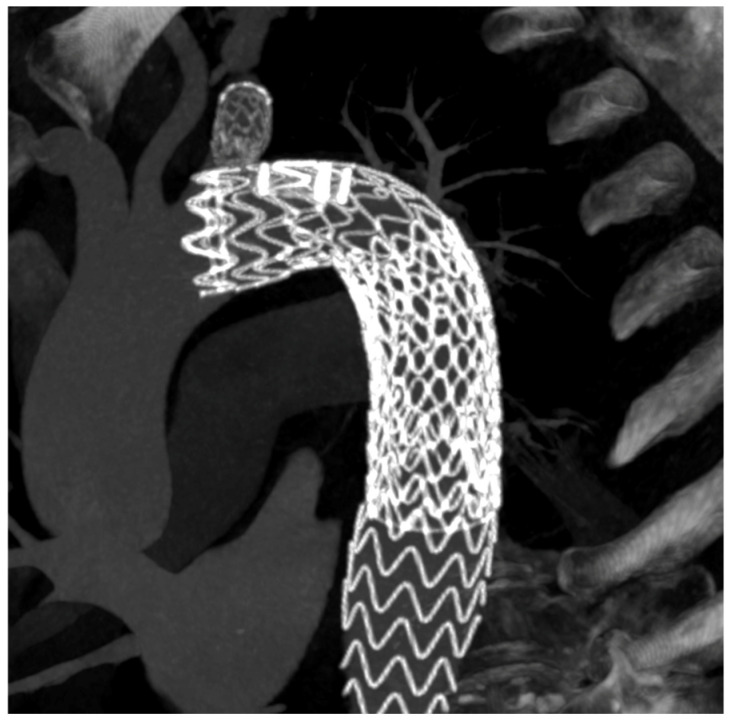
Postoperative 3D CT reconstruction after TBE implantation combined with a distal straight thoracic stent-graft module.
